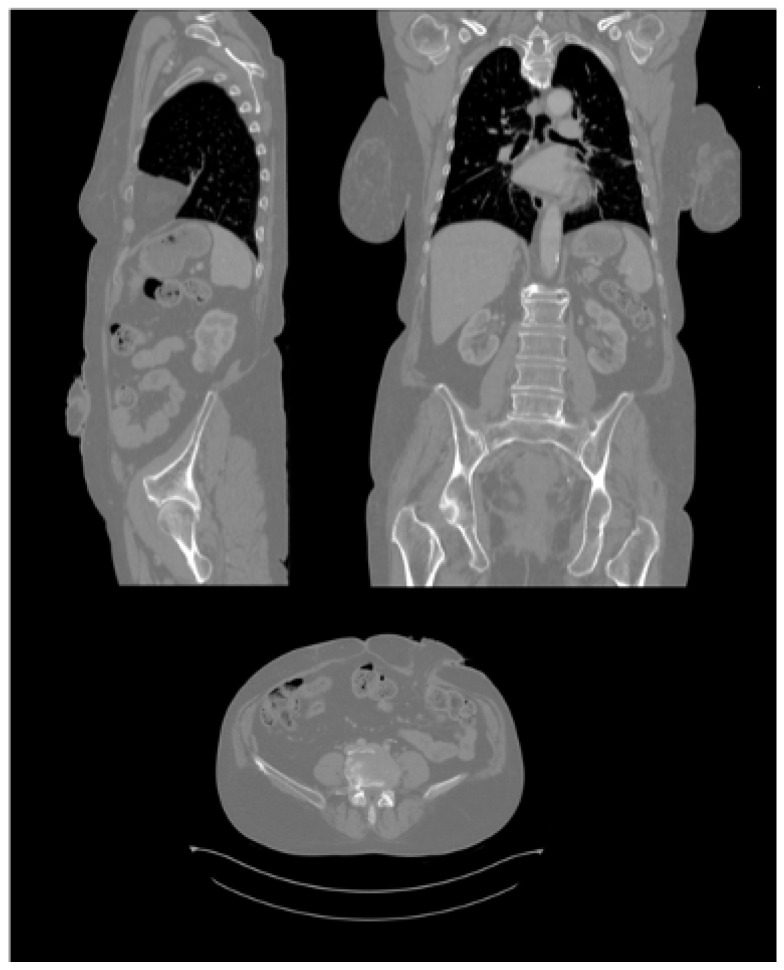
Cross sectional images (sagittal, coronal and axial) of an abdominal CT scan [31].
